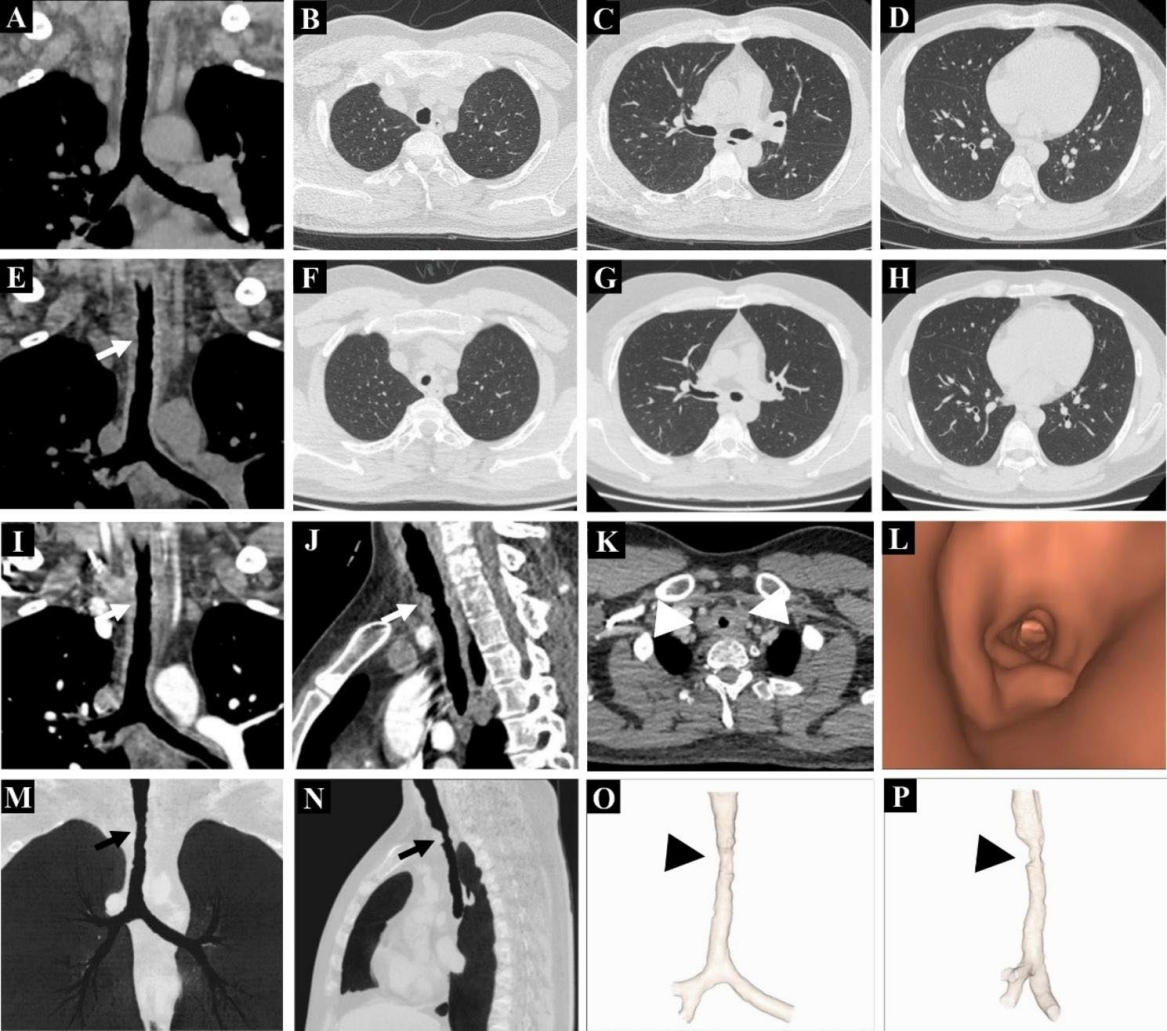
Chest high-resolution CT findings. (A-D) Four months ago, chest CT showed no abnormality. (E) Coronal CT showed diffuse thickening of the trachea and bilateral principal bronchus (white arrow). (F-H) Axial CT showed no focal findings in the lungs. (I-J) Coronal and sagittal enhanced CT showed obvious enhancement of tracheobronchial lesions (white arrow). (K) Axial enhanced CT showed slightly enlarged and enhanced lymph nodes in the upper mediastinum (white triangle arrows). (L) Virtual endoscopy showed irregular protuberant lesion of the trachea. (M-N) Minimum intensity projection showed tracheobronchial wall thickening resulted in different degrees narrowing of the tracheal lumen (black arrow). (O-P) Volume rendering technique showed tracheal stenosis of different degrees, especially in the upper and middle segments (black triangle arrow)
